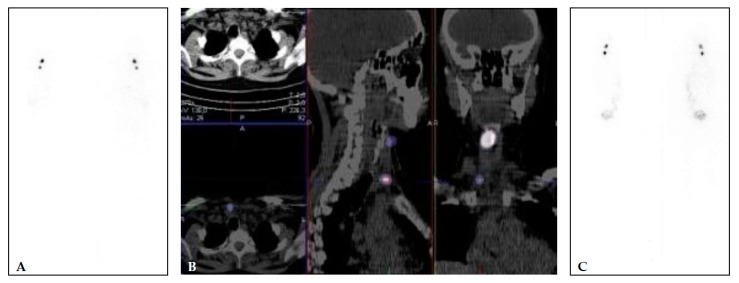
(A,B) 40 years old female, with multifocal papillary microcarcinoma (left lobe 7 mm and 2 foci of 1 mm in the right lobe (pT1a(m)N0). TSH 67.83 mIU/L, Tg 11.83 μg/L (<2 μg/L), and TgA 2.2 kIU/L (<4.5 kIU/L). Diagnostic WBS and SPECT/CT (A,B) performed after application of 74 MBq of [^131^I] show two focuses of [^131^I] uptake—larger in the right upper part of the region VI and small focus lower in the region VI, right paratracheal. The patient was treated with 1665 MBq (45 mCi) of [^131^I]. Post-treatment [^131^I] WBS (C) shows an accumulation of the [^131^I] in the same two focuses.
